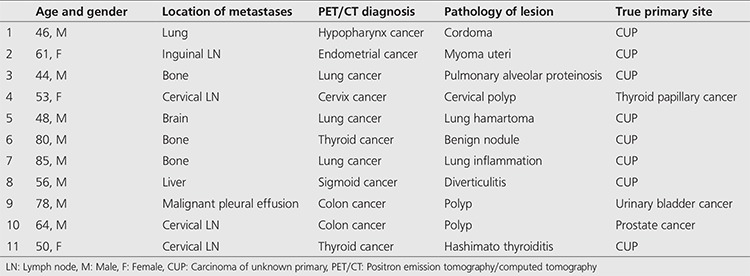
The eleven false-positive results diagnosed by ^18^F-fluorodeoxyglucose positron emission tomography/computed tomography
